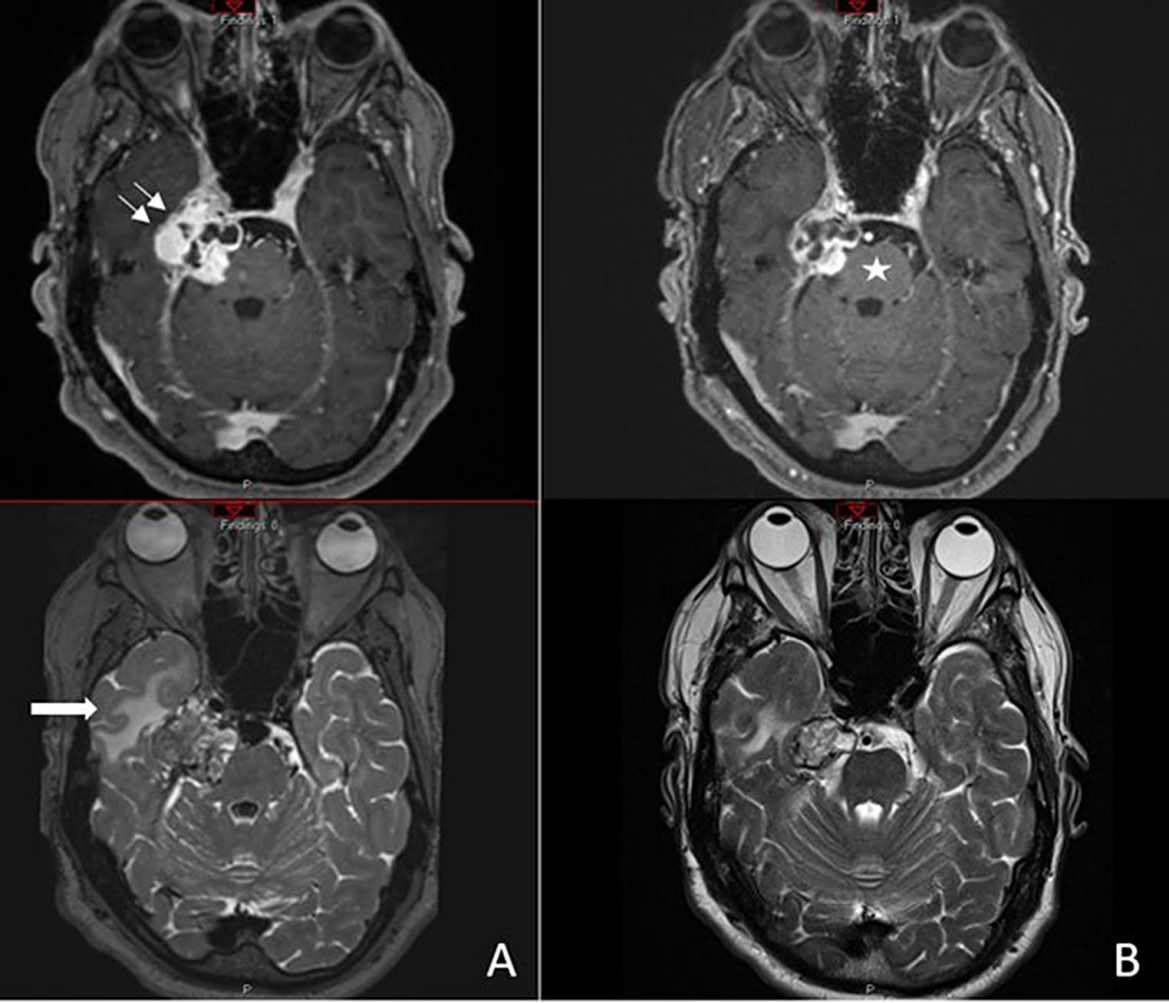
Brain MRI showing belzutifan-induced tumor reduction of sporadic hemangioblastoma of the right Meckel’s cave. (A) baseline MRI before starting belzutifan, (B) follow-up MRI after 9 months of belzutifan 80 mg daily treatment. (A and B) axial T1 post contrast (top) and axial T2 (bottom) images show: 1) a substantial decrease in the overall size of the lobulated, heterogeneously enhancing solid-cystic mass involving the right trigeminal nerve, the right cavernous sinus, and expanding the right Meckel’s cave (from 3.5 × 3.5 cm in A to 2.6 × 2.9 cm in B); 2) decreased size in the solid components (double white arrow); 3) decreased mass effect on the pons (white star) and improved right temporal edema (white arrow)
